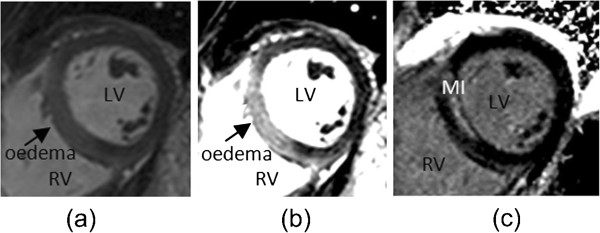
Example of short axis CMR images: (a) an initial bright blood T2-weighted oedema image and (b) transmural oedema more clearly revealed after user adjustment of window and level settings; (c) the corresponding LGE image reveals non-transmural hyperenhancement in the septum.
